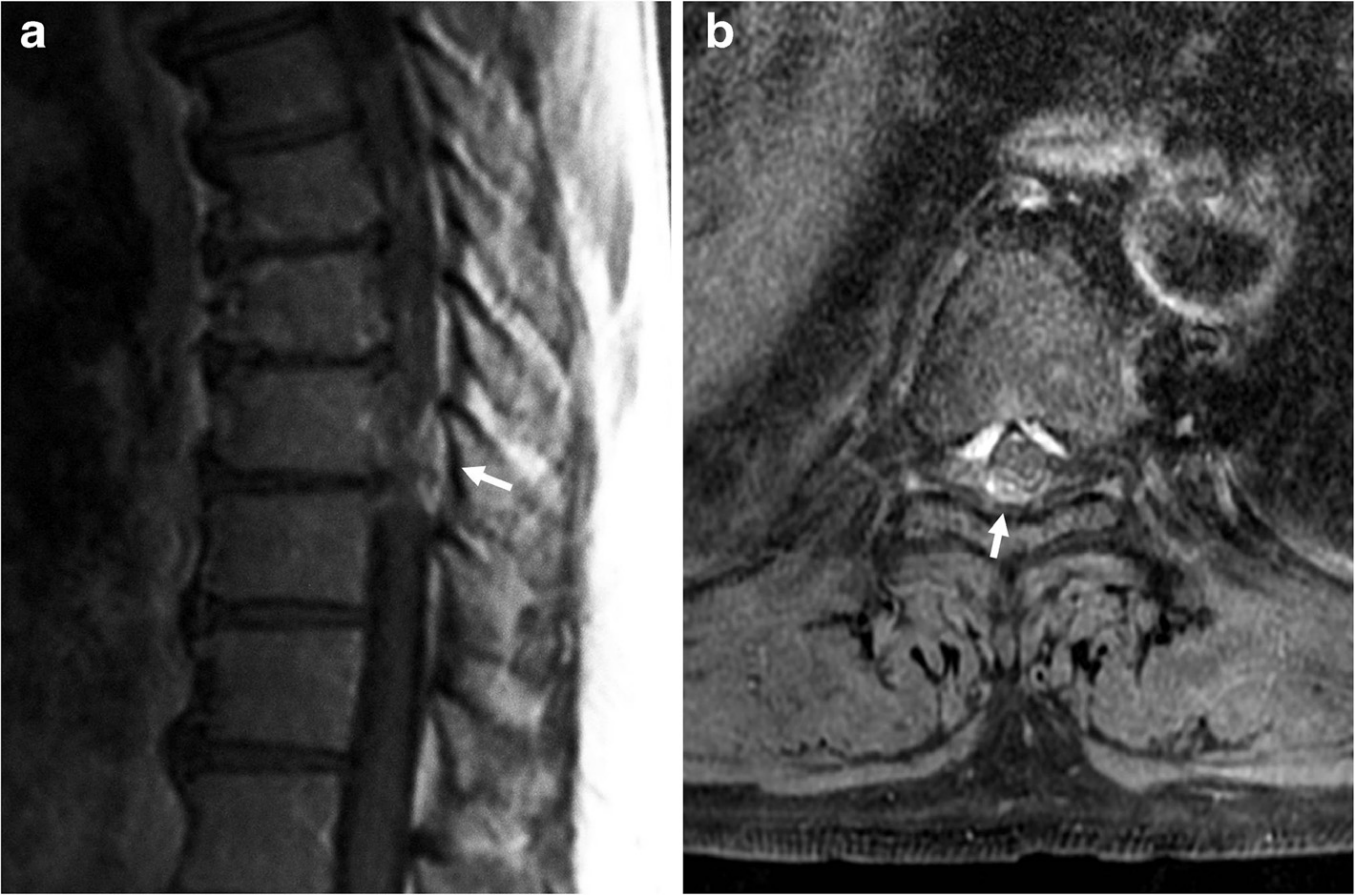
Pre-operative magnetic resonance imaging of the thoracic spine. Sagittal gadolinium-enhanced T1-weighted imaging (a) and axial gadolinium-enhanced T1-weighted imaging with fat suppression (b) show a peripherally and heterogeneously enhancing posterior epidural mass at the T9-T10 level, compressing the spinal cord (arrows).
